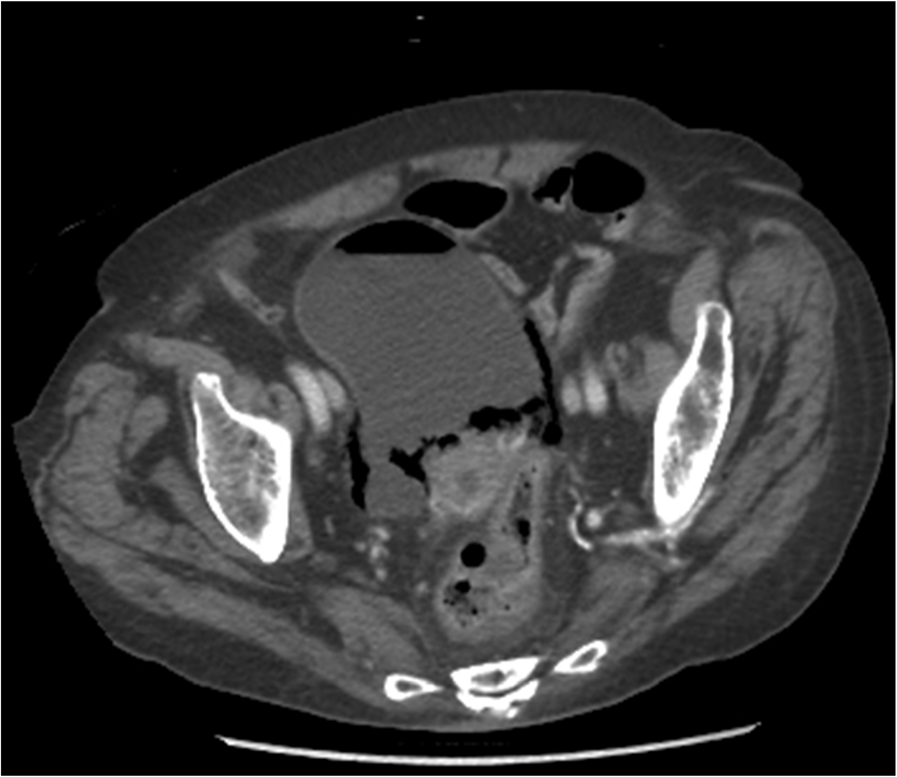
Computed tomography. Axial view showing intraluminal air and emphysematous changes of the bladder wall.
